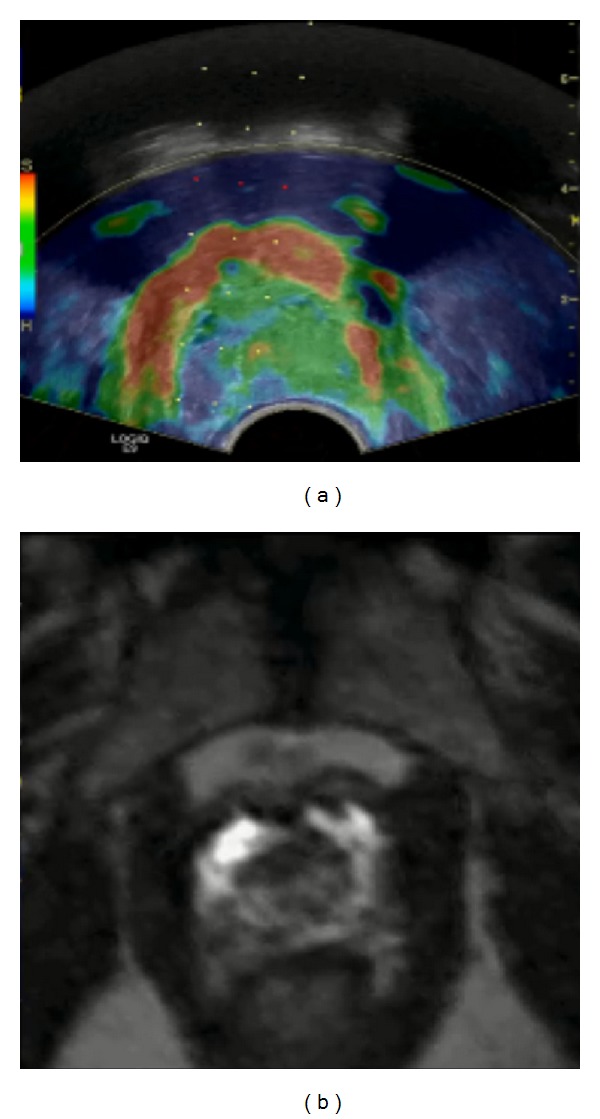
RTE demonstrates the low signal intensity nodule on T2-weighted image (b) as a lesion of decreased elasticity ((a), colour-coded blue).
